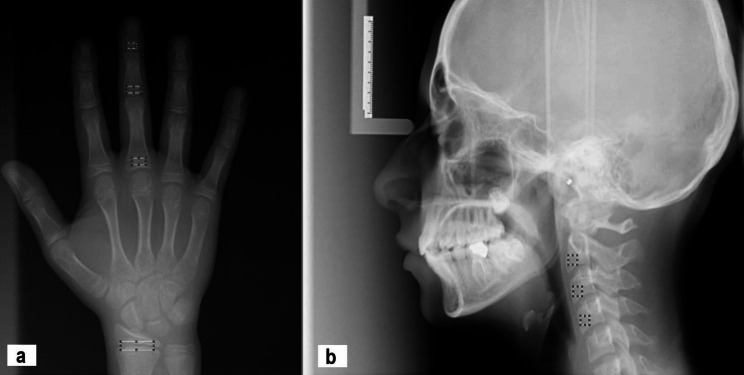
(a) Regions of interest (ROIs) from 4 different sites on hand-wrist radiographs (proximal, distal, medial phalanx and radius), (b) ROIs from 3 different sites on the cephalometric image (C2, C3 and C4 cervical vertebral bodies)
